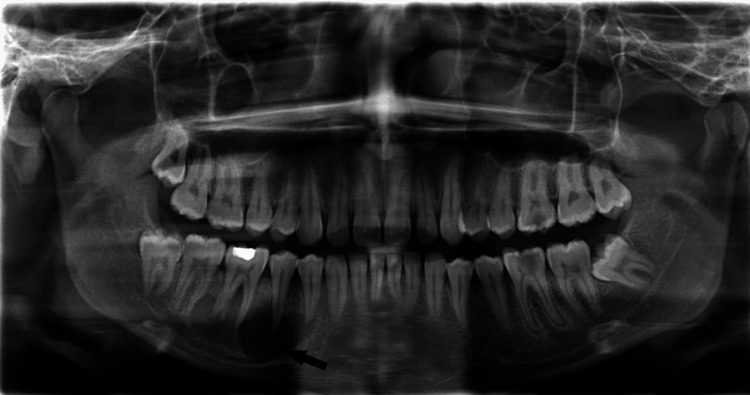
OPG showing a well-defined radiolucent periapical lesion associated with right mandibular premolars and first molar. OPG- Orthopantamogram
